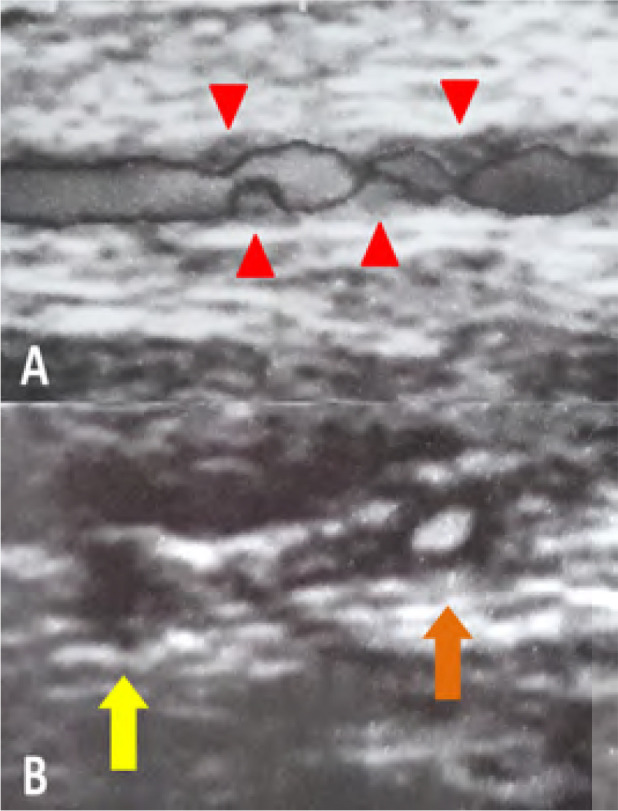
Duplex ultrasonography of superficial common temporal arteries and their main branches in longitudinal (A) and transverse (B) scan. Hypoechoic areas in the vessel wall are shown in the right superficial common artery (A: red arrowheads). A “halo sign” is seen in the parietal branch of the left temporal artery (B: brown arrow), while no blood flow can be detected in the frontal branch (B, yellow arrow).
